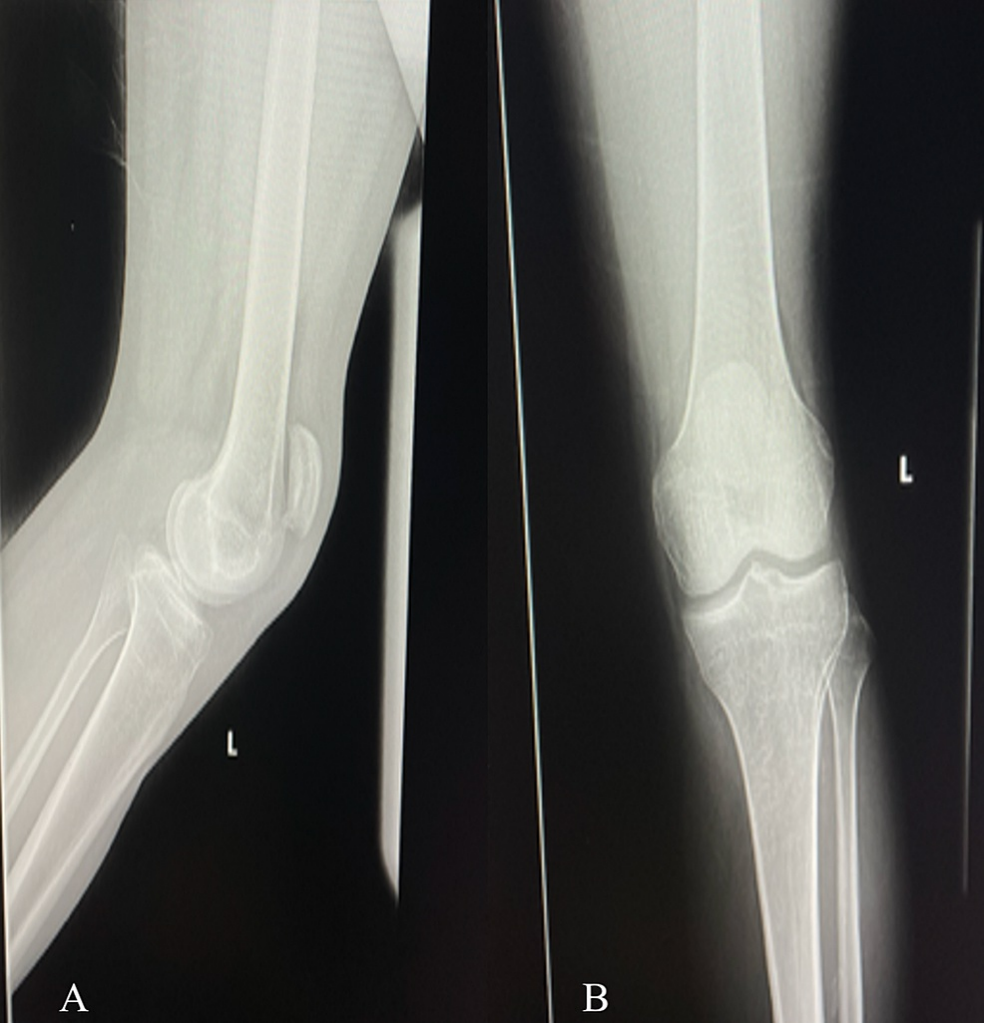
Radiograph of the left knee: (A) lateral and (B) anteroposterior view showing the high-riding patella.
